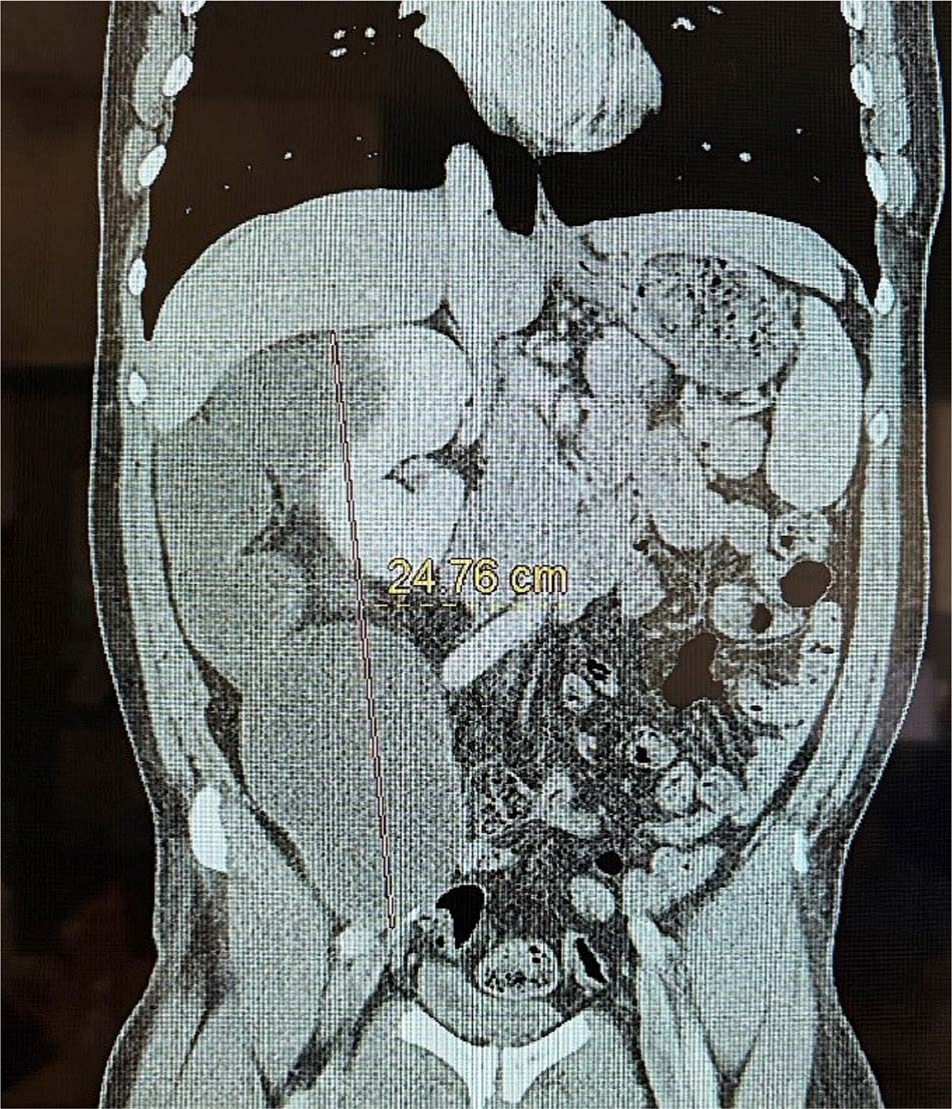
Coronal CT of the mucinous adenocarcinoma.
